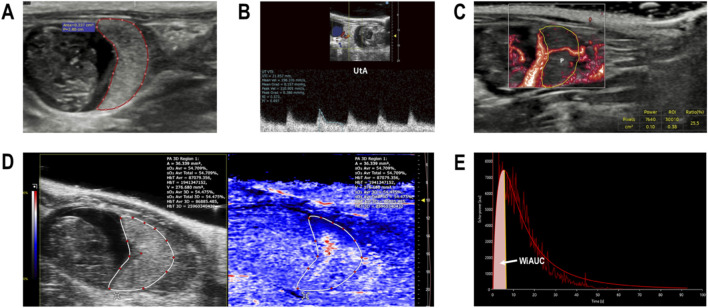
Representative images of longitudinal multimodal placental assessment. (A) B-mode ultrasound for the morphological assessment of maximal placental cross-sectional area (red outline). (B) Uterine artery (UtA) pulse-wave Doppler waveforms used for resistance index (RI) calculation. (C) MV-flow imaging visualizing microvascular flow signals (vascular index, VI). (D) Photoacoustic imaging (PAI) map showing the distribution of hemoglobin oxygen saturation (sO_2_). (E) Representative contrast-enhanced ultrasound (CEUS) time-intensity curve (TIC). Red line: fitted model; shaded area: wash-in area under the curve (WiAUC), serving as a surrogate for relative microvascular blood volume.
